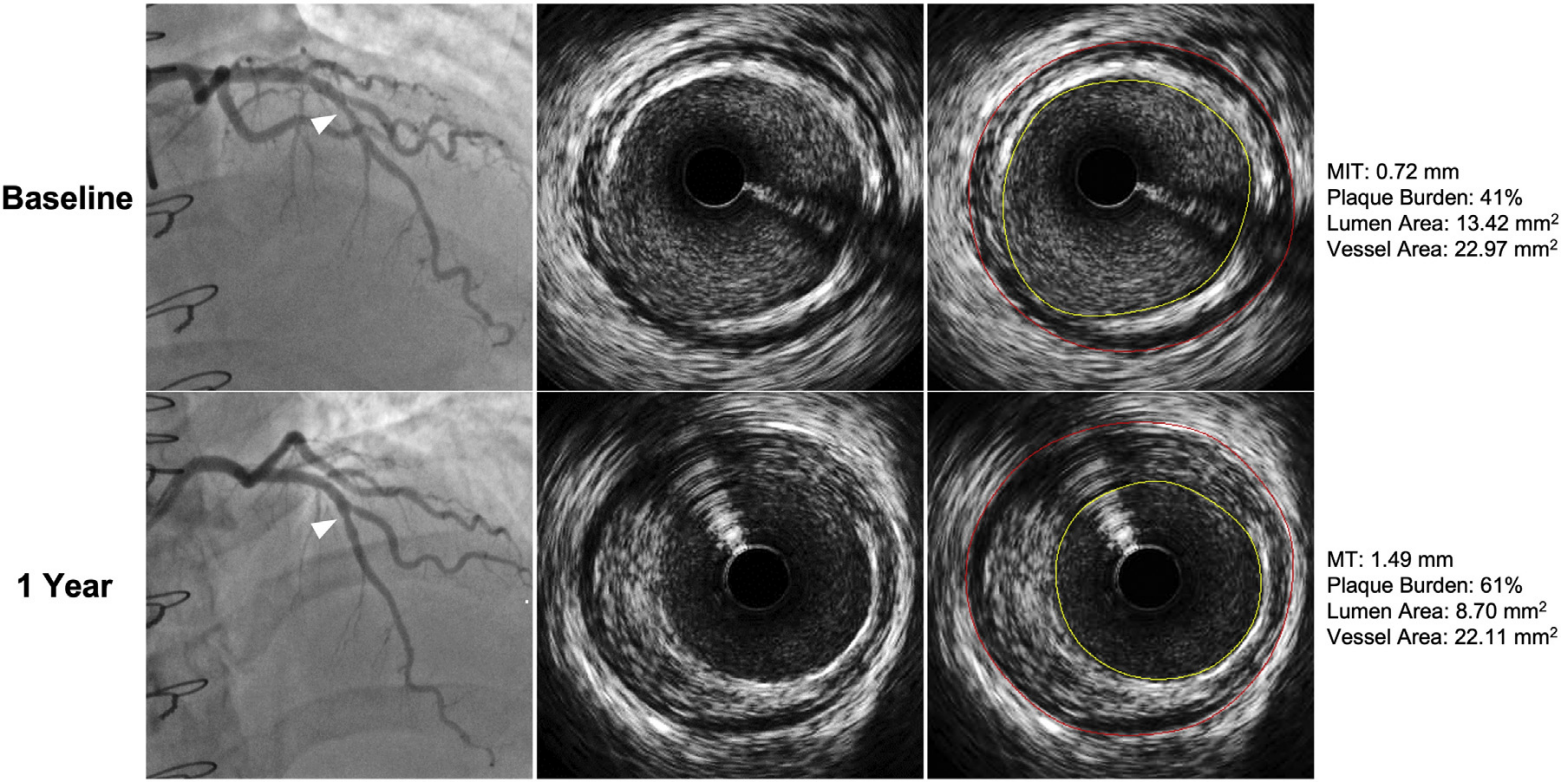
Paradoxical remodeling demonstrated by IVUS 1 ​year after heart transplantation. Serial IVUS cross-sections in a mild stenosis (arrowhead) of the LAD obtained at baseline (upper panels) and 1 ​year (lower panels) after heart transplantation. Maximal intimal thickness (MIT) increased by 0.77 ​mm in a year without compensatory (adaptive) vessel remodeling (ie, paradoxical remodeling). IVUS, intravascular ultrasound; LAD, left anterior descending.
